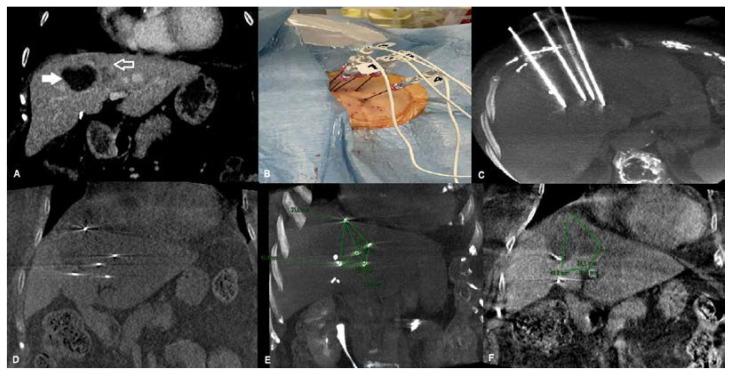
An 81-year-old female affected by recurrence of liver metastasis from pancreatic cancer after percutaneous ablation and SBRT, treated with percutaneous ECT. (A) Pre-treatment coronal CT reconstruction showing dishomogeneous enhancing tissue consistent with metastatic liver recurrence (contoured arrow) at the periphery of the hypodense post-ablation and post-SBRT area (white arrow). (B) Percutaneous placement of the five electrodes. (C) Unenhanced cone-beam CT MPR and MIP reconstruction showing the five electrodes positioned in the target lesion. (D) Unenhanced cone-beam CT coronal reconstruction showing the tip of the five electrodes in place. (E) Unenhanced cone-beam CT coronal reconstruction showing the tip of the five electrodes in place and the post-processing measurements underlining the geometrical relationship between each electrode. (F) Contrast-enhanced post-ECT cone-beam CT coronal reconstruction showing the ablation area. Legend: ECT: Electrochemotherapy; SBRT: Stereotactic body radiation therapy; CT: Computed tomography; MPR: Multiplanar reconstruction; MIP: Maximum intensity projection.
